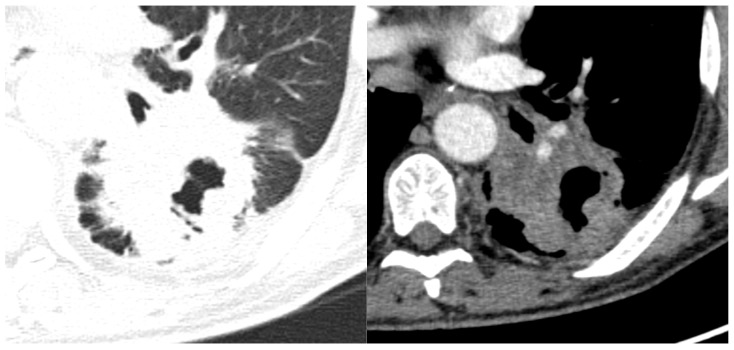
Axial CT of a peripheral SQCC with intratumoral cavitary lesion with anfractuous border; pleural invasion through spiculated processes which have no with the posterior costal arch can be observed.
